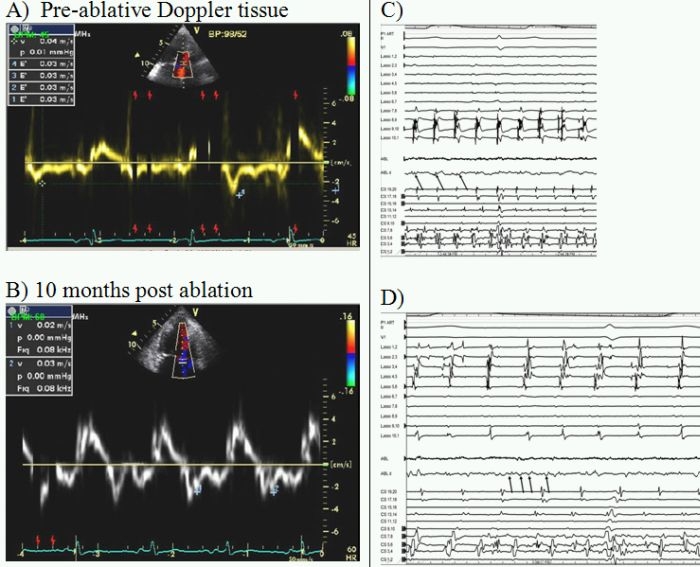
A pre-ablation tissue Doppler velocity increasing showing severe diastolic dysfunction (e' 3 cm/s) findings were virtually unchanged 10 months post ablation (B) with e' 3 cm/s,  LAVI of 82 ml/m^2^ and LVEF 44%. C/D show representative intracardiac atrial electrograms pre- and post-ablation respectively.  Marked electrogram fragmentation with high frequency signals suggesting of advanced secondary atrial substrate disease that correlated with echo based prediction.
